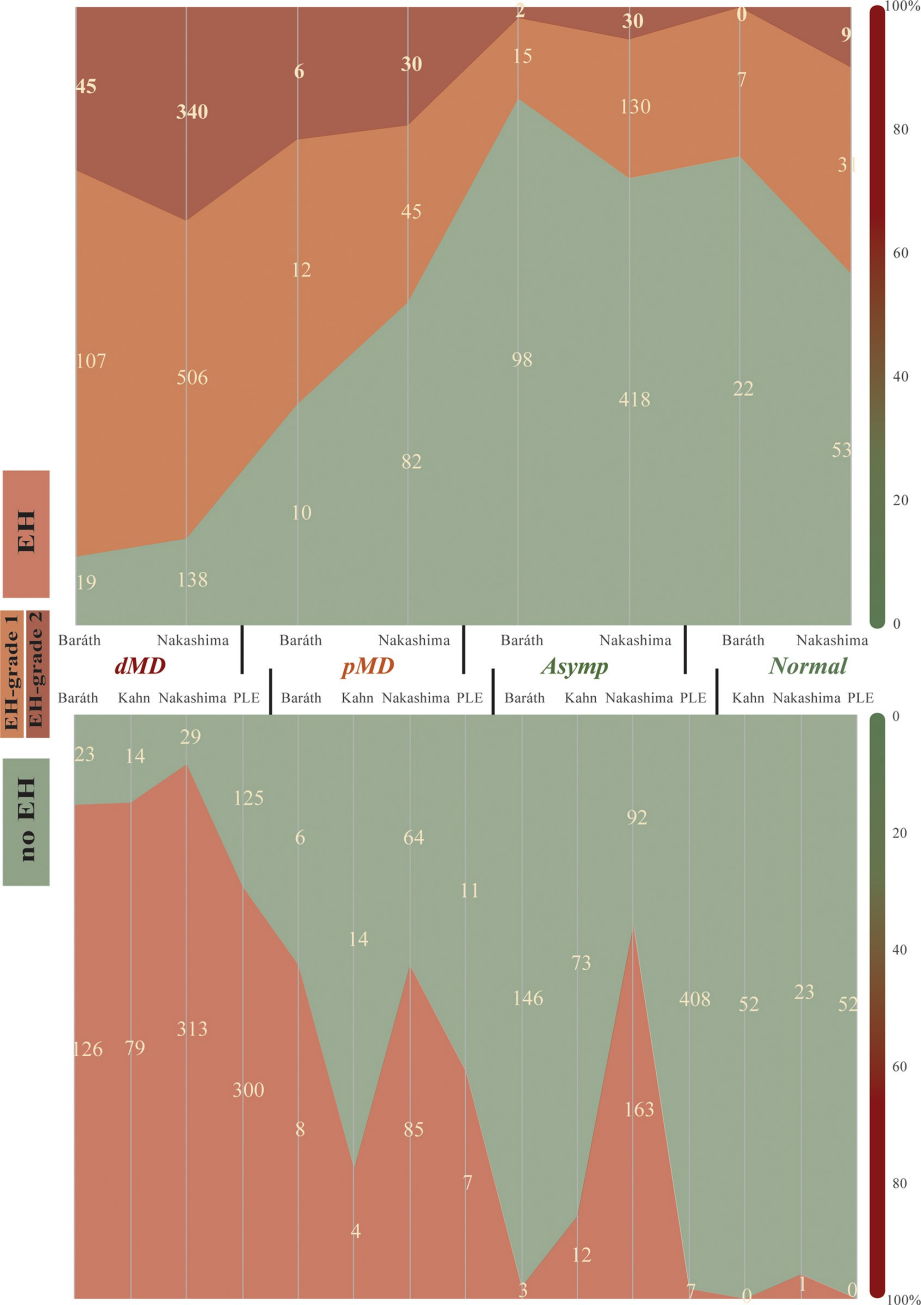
MRI-based cochlear hydrops grading and PLE in MD.
